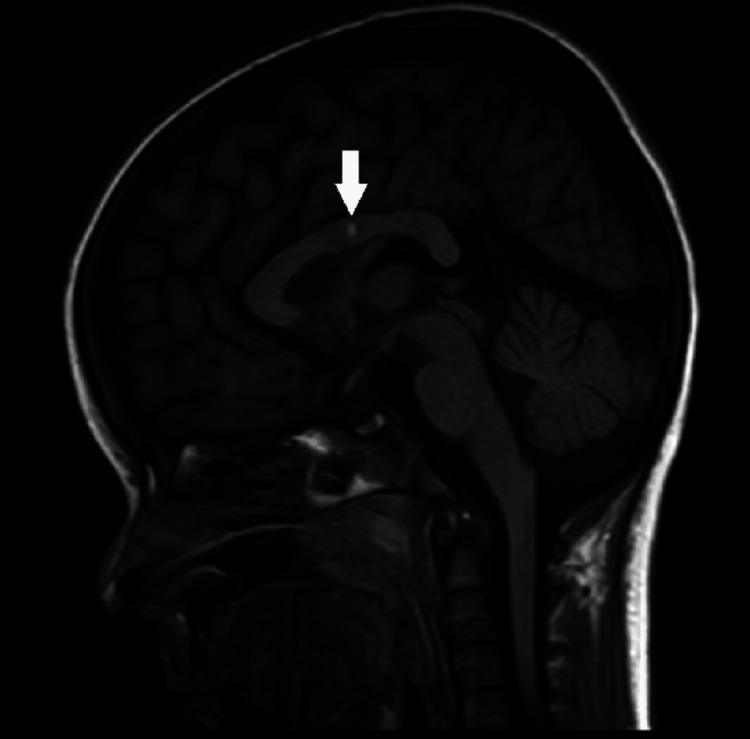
MRI brain non-contrast T1WI sagittal reformatted sequence demonstrating focus of T1 hyperintensity in the body of corpus callosum (white arrow) suggestive of hemorrhagic nature of infarct of corpus callosum
